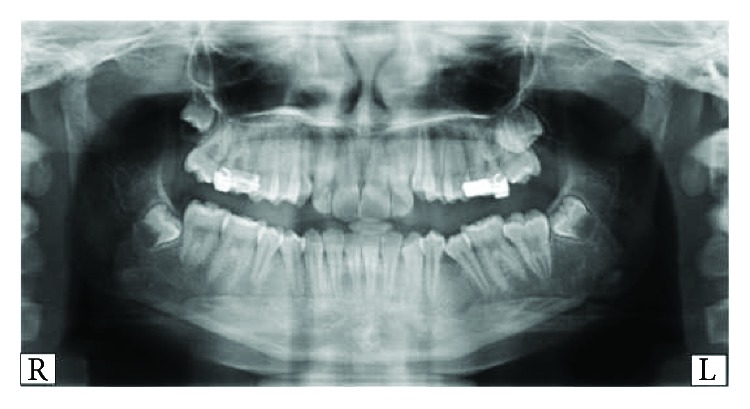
Orthopantomogram (OPG) showing the absence of any radiolucent lesion and the successful eruption of the mandibular left second premolar.
